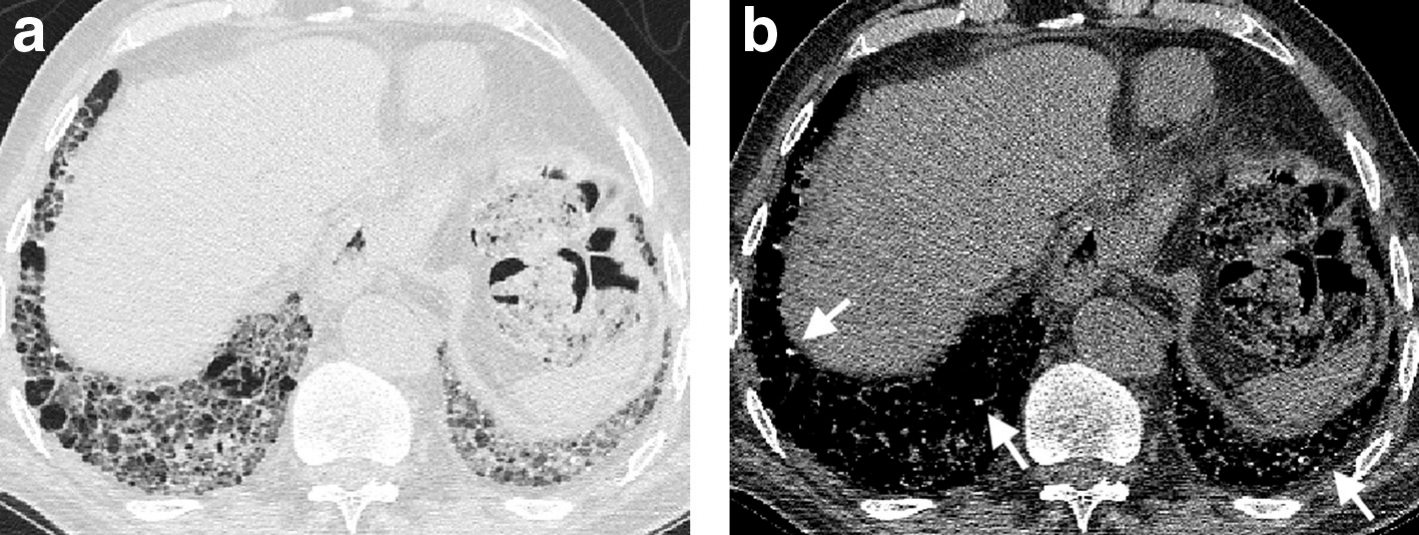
Nodular areas of parenchymal ossification (arrows) seen on axial CT images in the right lower lobe in a patient with idiopathic pulmonary fibrosis. Images are shown on lung windows (a) and mediastinal windows (b).
